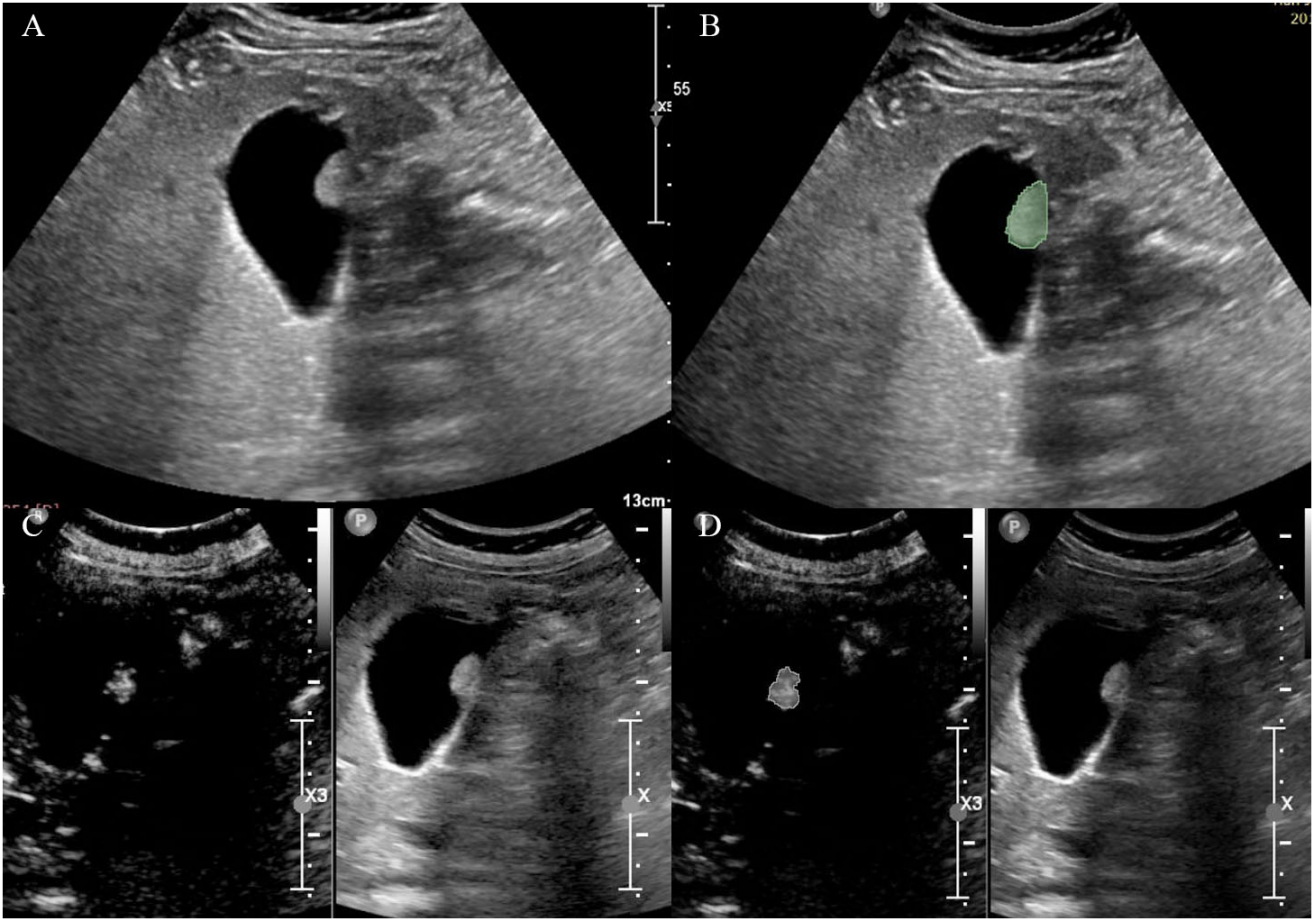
Delineation of the region of interest on (A, B) grey-scale ultrasound and (C, D) CEUS images.
